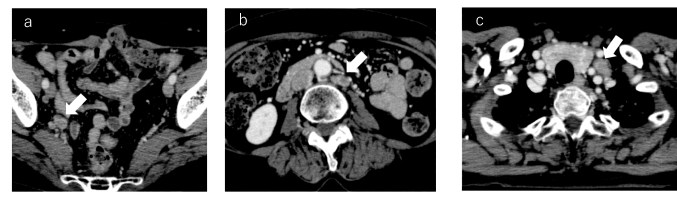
Contrast-enhanced CT images from a 60-year-old woman with stage IC clear cell carcinoma. a Right internal iliac lymph node metastasis (arrow) was detected 14 months after surgery. b Subsequently, para-aortic lymph node metastasis (arrow) and c left supraclavicular lymph node metastasis (arrow) were found 36 months after surgery
